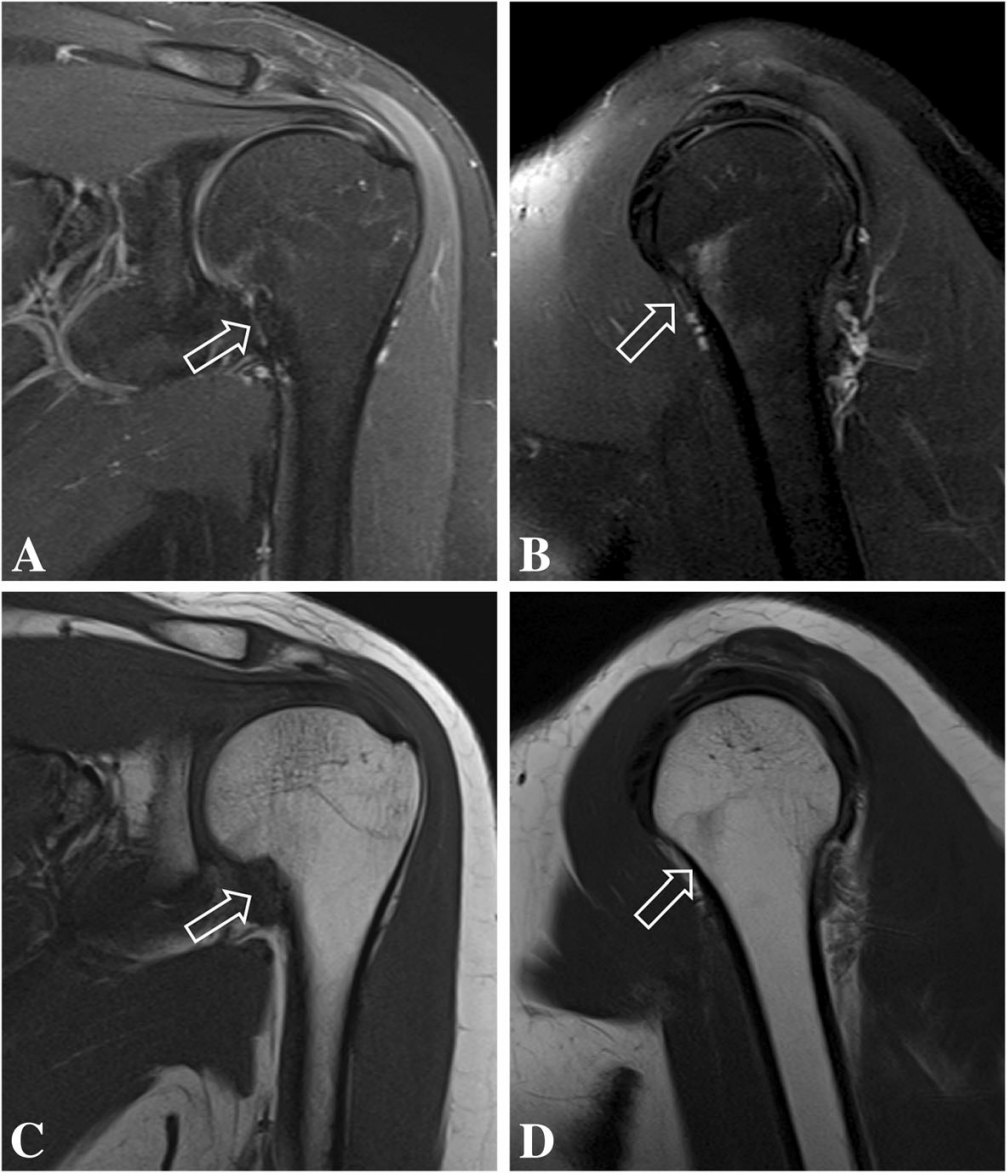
Coronal (a) and sagittal (b) T2-weighted fat-suppressed follow-up MR images and coronal (c) and sagittal (d) T1-weighted MR images of the left shoulder showing resolution of the bone marrow edema of the proximal humerus (open arrow)
